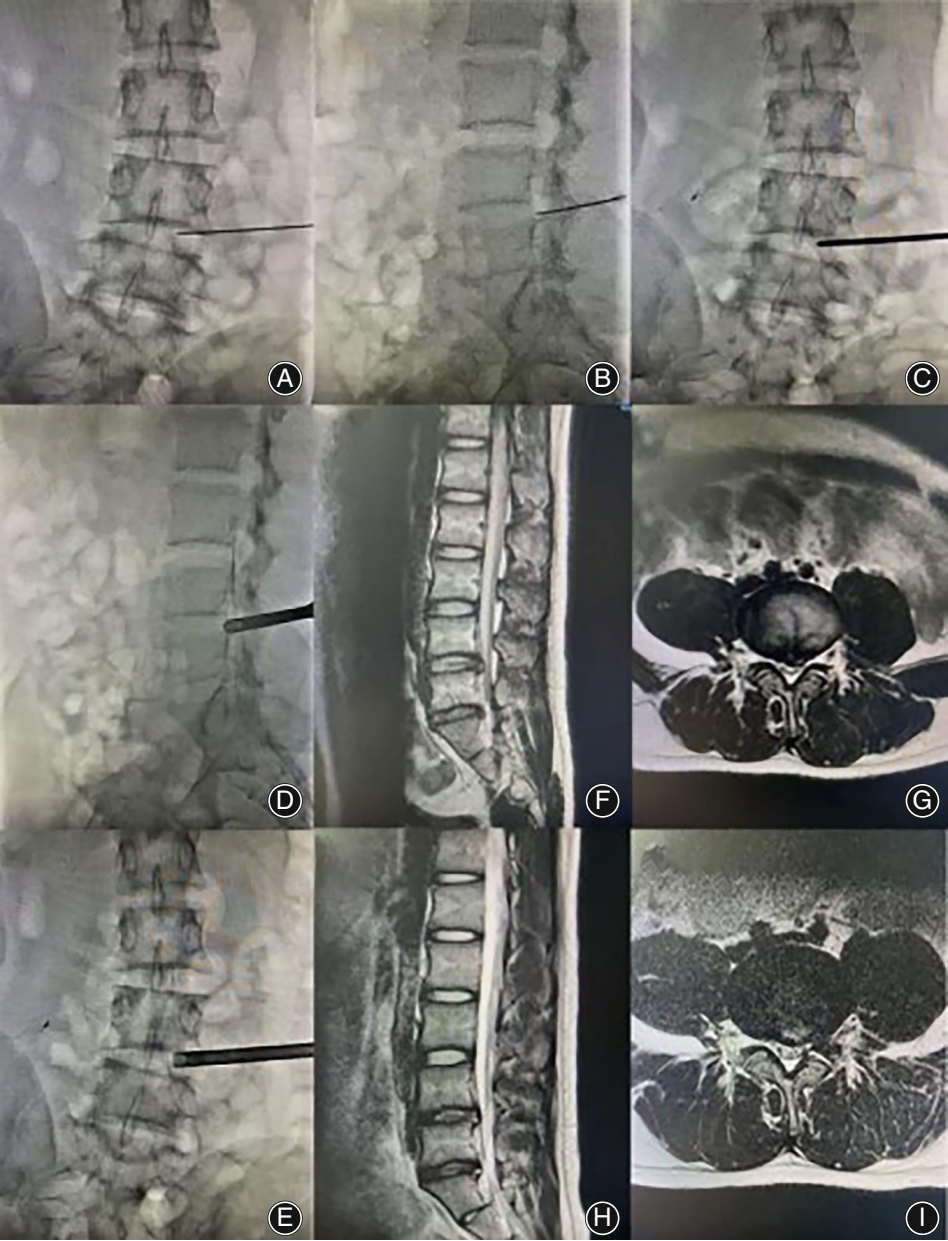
PETD intraoperative fluoroscopy and preoperative and postoperative. MRI (A–E) Intraoperative radiographs. (F and G) Preoperative MRI, H and I: MRI 3 months after surgery.
